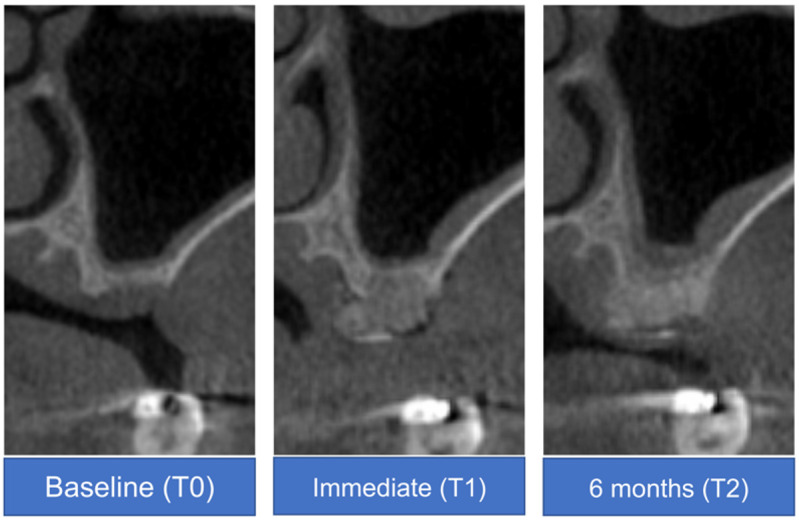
Cone-beam computed tomography analysis of a representative case at baseline (T0), immediately after surgery (T1), and at 6 months after surgery (T2).
